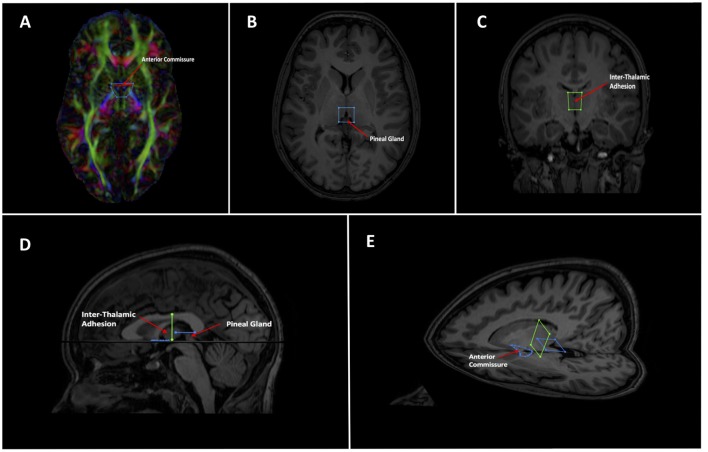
Showing initial gate placement. (A) Anterior SEED gate placement: the most superior point of the anterior commissure is found on a First eigenvector fractional anisotropy (FEFA) axial DWI slice. The anterior boundary of the SEED gate is placed just anterior to the commissure and the gate is extended broadly posteriorly past the columns of the fornix. The anterior commissure is labeled. (B) Posterior SEED gate placement: In an axial T1 image, the slice *above* the most superior visible part of the pineal gland is chosen. The anterior boundary of the SEED gate is placed approximately at the mid thalamus and extended to where the mid pineal is found on the slice below. The gate should extend laterally to cover the approximate stalks of the pineal gland. Note that the pineal *is visible* in this image, as this is taken to show the pineal for localization purposes. The pineal is labeled. (C) Mid AND gate placement: the mid-point of the thalamus (using the inter-thalamic adhesion if present) is found on a coronal T1 image slice. The superior boundary of the AND gate is placed above the thalamus at the lower level of the fornix and extended to the lower thalamus, making sure to encompass the inter-thalamic adhesion if present. The gate should be wide enough to cover most of the “curve” of the thalamus. The inter-thalamic adhesion is labeled. (D) Sagittal and (E) axial-sagittal views of the initial SEED and AND gates. The anatomical fixed points for gate placement: anterior commissure, inter-thalamic adhesion and pineal gland are labeled.
